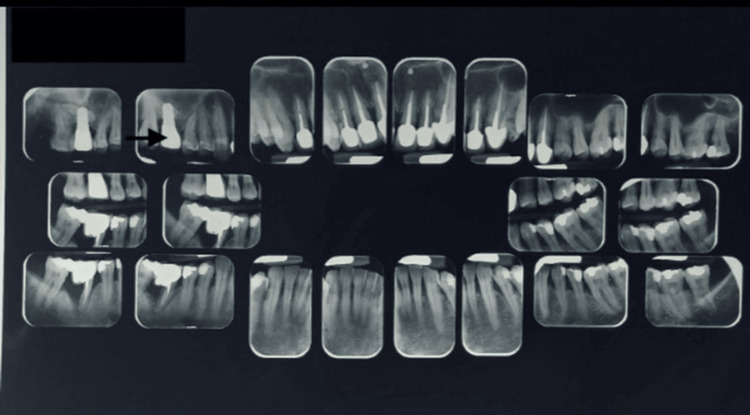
View at 27-month follow-up: full-mouth long-cone periapical radiographic survey (arrow), following biannual scaling and root planing
